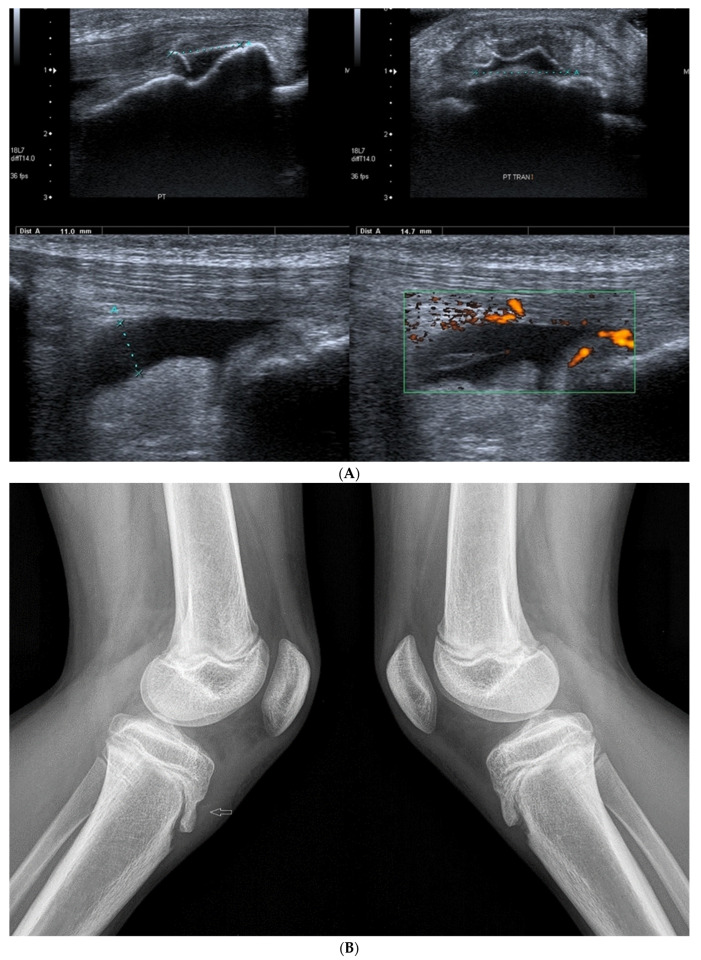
(A) The case of a 13-year-old girl in whom the symptoms of Osgood–Schlatter disease were clearly related to a sport injury (avulsion of the bony fragment from the tibial tuberosity). At the top, there are scans, longitudinal on the left and transverse on the right, with a marked thin layer of bone detached from the surface of the tibial tuberosity. At the bottom, two scans in the longitudinal section where there is an exudate in a deep subpatellar bursa. On the right, there is increased vascularization of the adjacent tissues (Power Doppler). Linear probe 7–18 MHz. (B) Lateral X-ray images of the patient from Figure 9A On the left—affected left knee joint, the arrow marks a small bony fragment detached from the tibial tuberosity. On the right—a healthy right knee joint.
